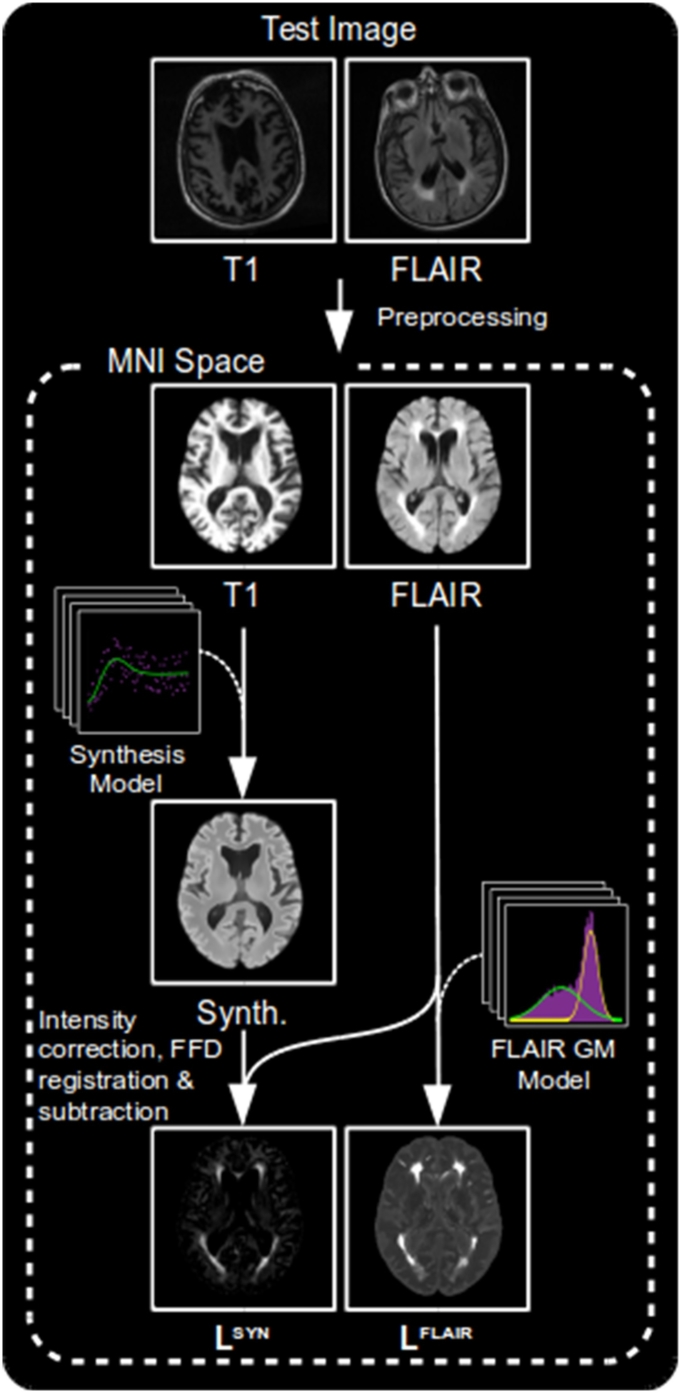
An overview of the process of creating the L^SYN^ and L^FLAIR^ likelihood maps.
